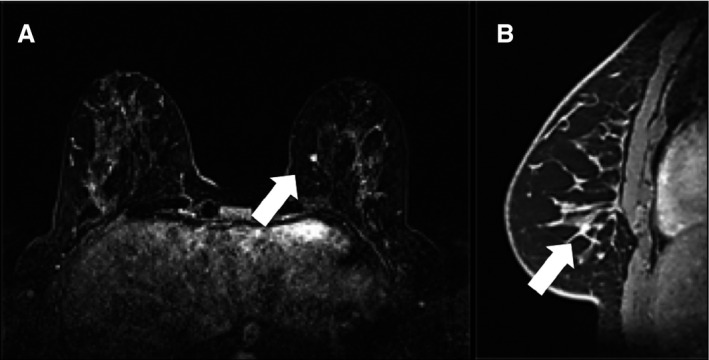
Preoperative breast MRI of a 52 years‐old woman, without family history of breast cancer with a newly diagnosed right breast cancer (not seen on the images). (A) Dynamic contrast‐enhanced breast MRI on the axial plane with postprocessed subtracted T1‐weighted images with fat saturation obtained 2 min after contrast injection and (B) Dynamic contrast‐enhanced breast MRI with postprocessed sagittal plane of subtracted T1‐weighted images with fat saturation obtained 2 min after contrast injection showing the focus of enhancement in the inner part of the left breast.
